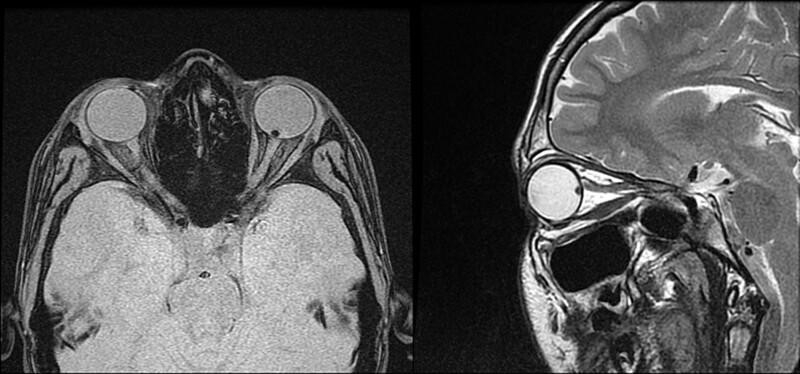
Magnetic resonance imaging of the head and orbits.
